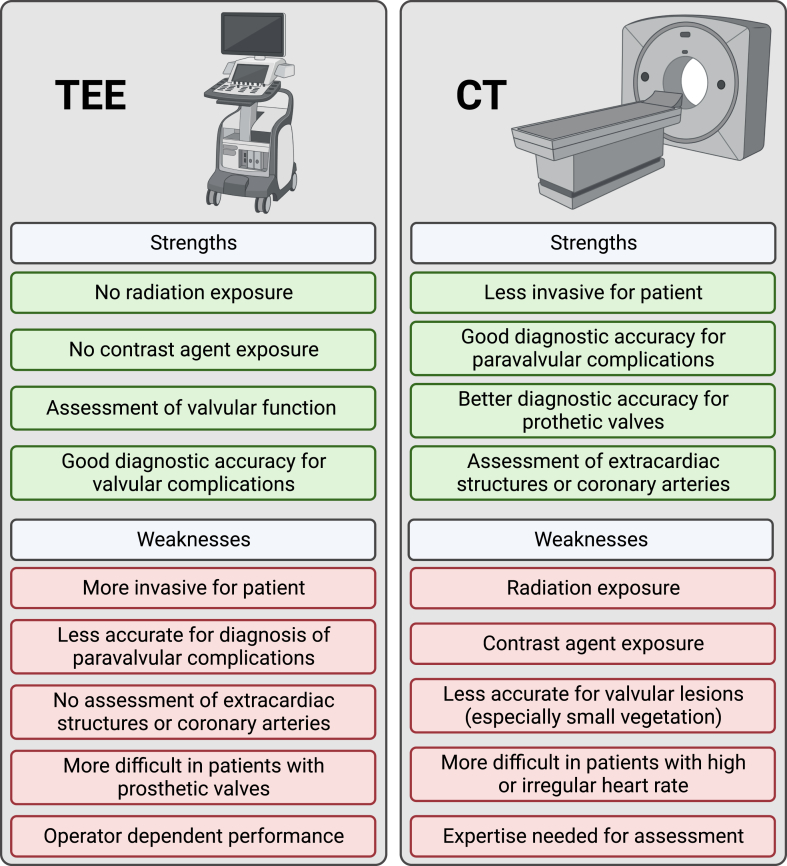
Overview of strengths and weaknesses of transesophageal echocardiography (TEE) and computed tomography (CT).
